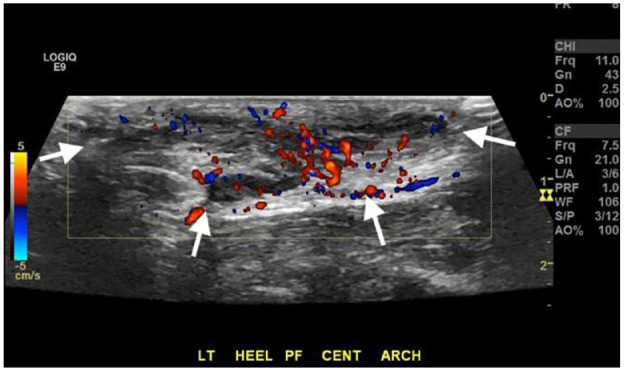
Static longitudinal sonographic image of the palpable 4.1-cm plantar foot mass (white arrows) located within the central band of the plantar fascia with associated hyperemia as seen with color Doppler, imaged prior to therapeutic collagenase (Xiaflex) injection.
